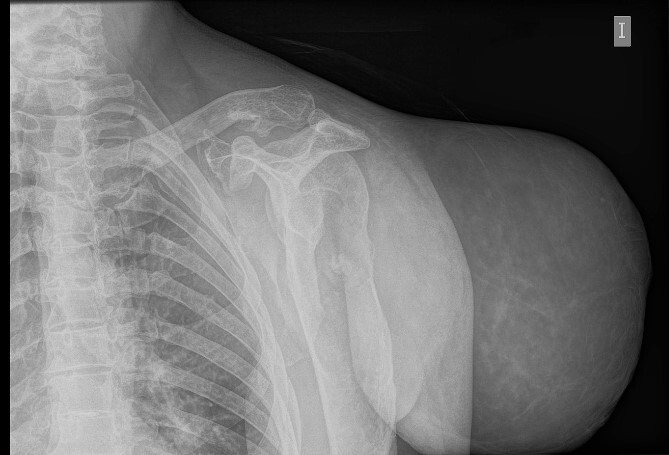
Simple radiography: evident soft tissue tumour on the left shoulder with multiple bone deformities compatible with osteochondromatosis.
